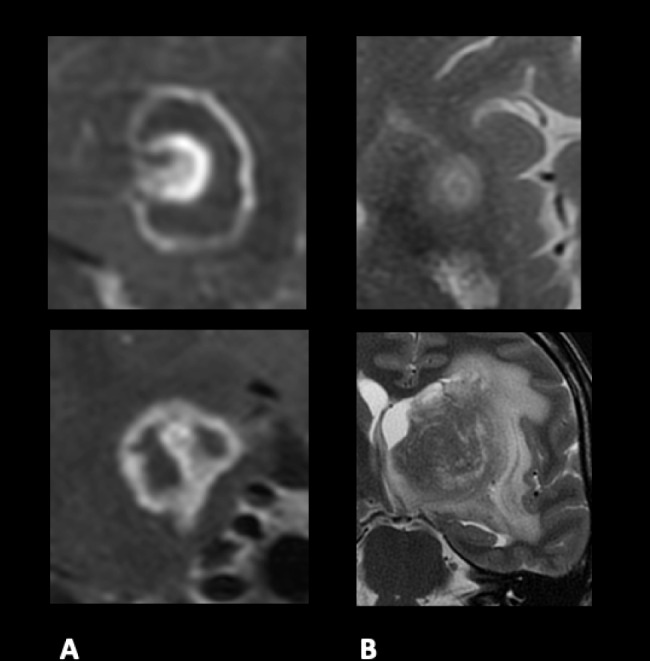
Examples of “eccentric” and “concentric” target signs in EBV-associated brain lymphoma. A “Eccentric target” signs on contrast-enhanced T1-weighted sequences; B “Concentric target” sign on T2-weighted sequences
